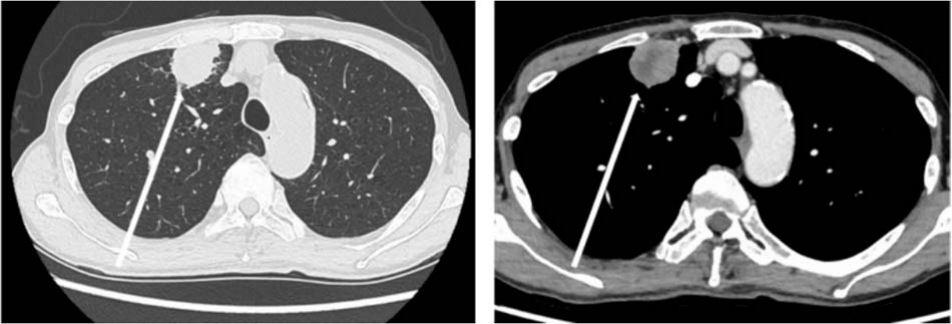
Chest computed tomography (CT) findings. An irregular mass 43 × 33 × 28 mm in size was found in the upper lobe of the right lung, which was suspected to be primary lung cancer.
